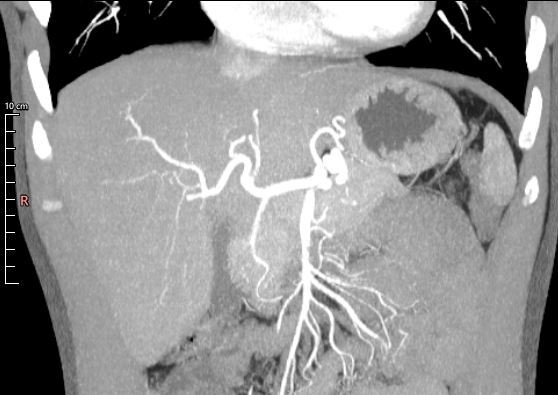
Abdominal computed tomography image of the abdomen reconstructed in maximum intensity projection in the arterial phase, coronal plane, showing a proper hepatic artery originating from a common hepatic artery and bifurcating into right and left hepatic arteries.
